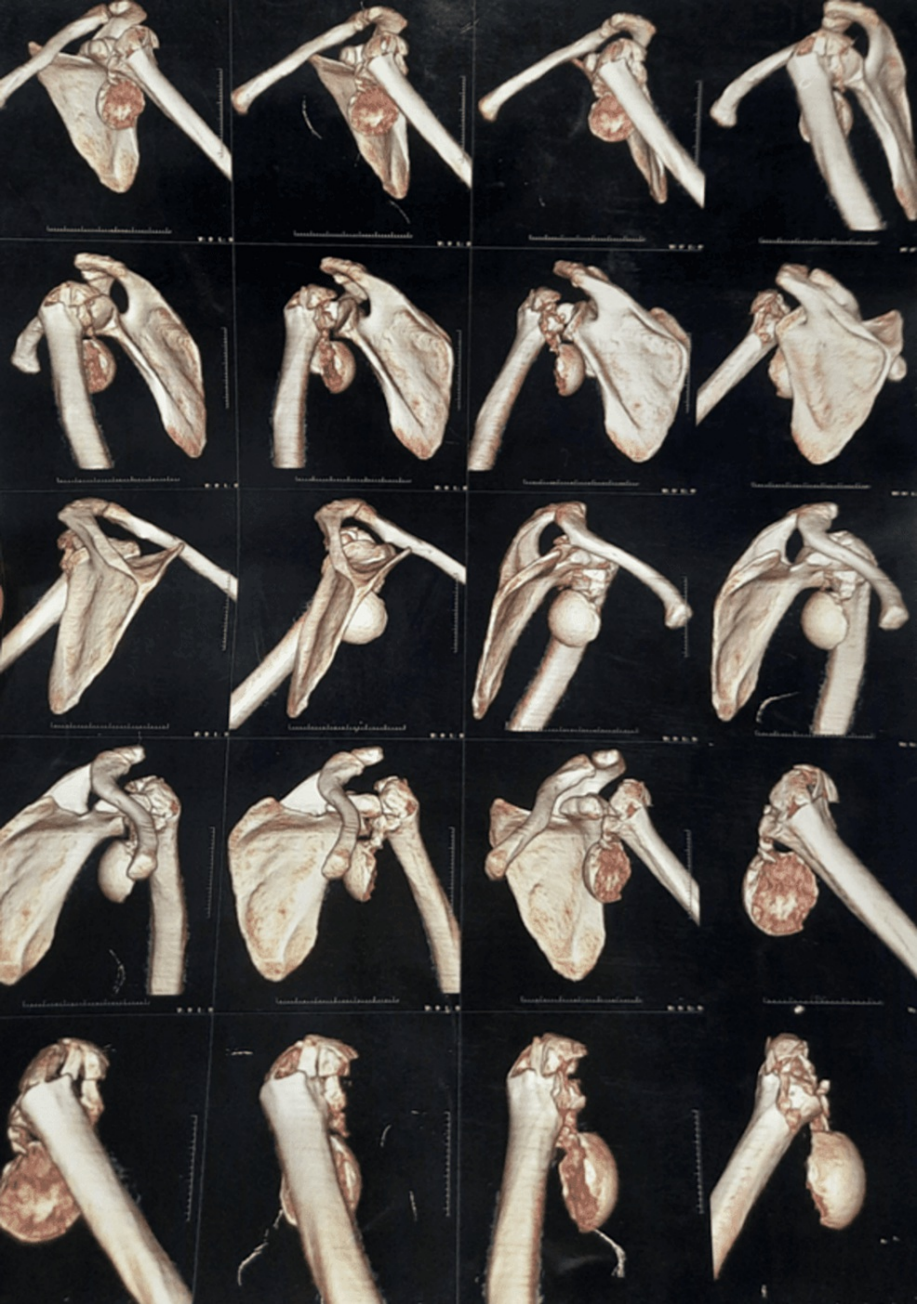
CT scan showing fracture and dislocation of left shoulder joint.
